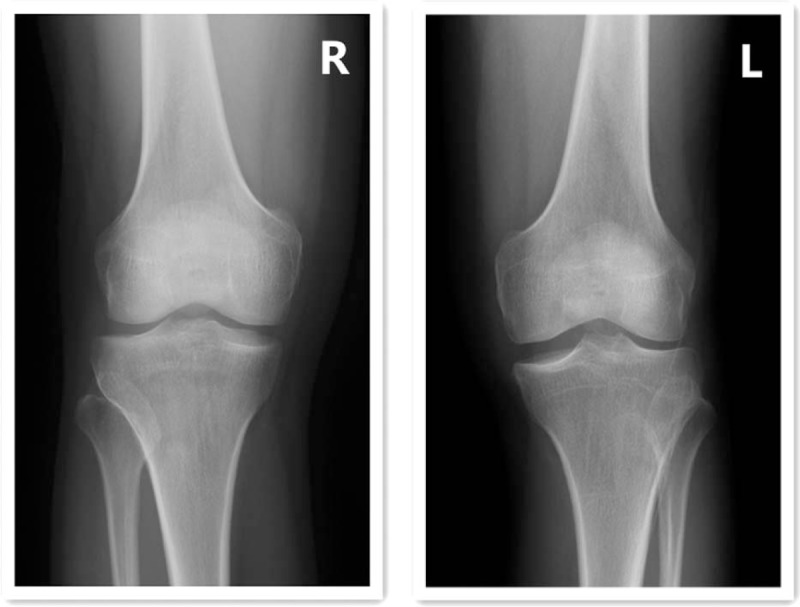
Standing anteroposterior radiographs of both knees. Radiography showed widened medial joint spaces, squaring of the medial femoral condyles, and cupping of the medial tibial plateaus bilaterally.
